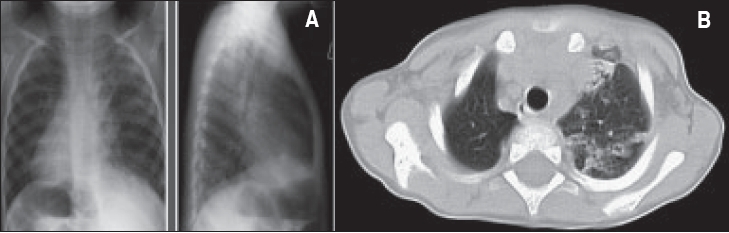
A patient with Karatgener syndrome and bilateral bronchiectasis 3A- Chest X-ray: Dextro cardia, right sided aortic arch. Diffuse air space disease through both lungs. Areas of hyper inflated lung between involved parts, which is typical of chronic lung disease. B. CT chest: Dextrocardia with inversion of abdominal viscera (situs inversus). Extensive chronic lung disease predominantly in the left lung with volume loss in the LUL, with patchy areas of atelectasis and diffuse bronchial dilatation.
